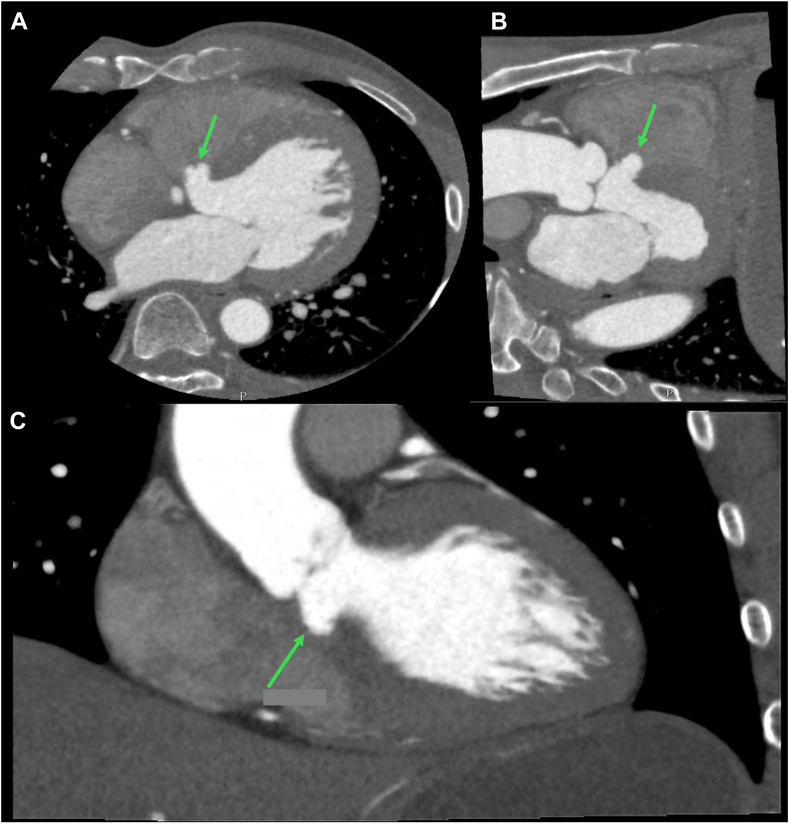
Cardiac computed tomography, multiplanar reconstruction (0.5 mm slice; 75% R-R), oblique axial (A), sagittal (B) and coronal (C), demonstrates normal cardiac dimensions and a saccular outpouching arising from the membranous septum with a narrow neck adjacent to the aortic annulus and tricuspid septal leaflet projecting toward the right ventricle, consistent with an IVMSA (*arrows*).
